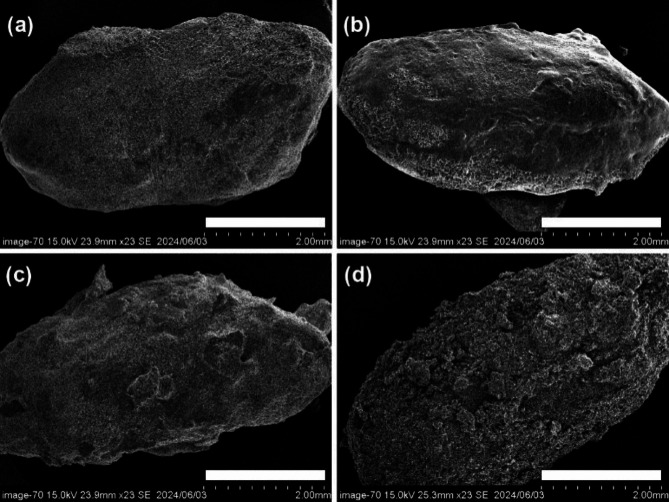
Morphological attributes of intact rice grains added with different supplements. (a) Normal cooked rice (CT); (b) cooked rice added with rapeseed oil (RRS); (c) cooked rice added with dried chili pepper powder (CRS); (d) cooked rice added with dried wasabi powder (WRS), scale bars indicate 2 mm.
